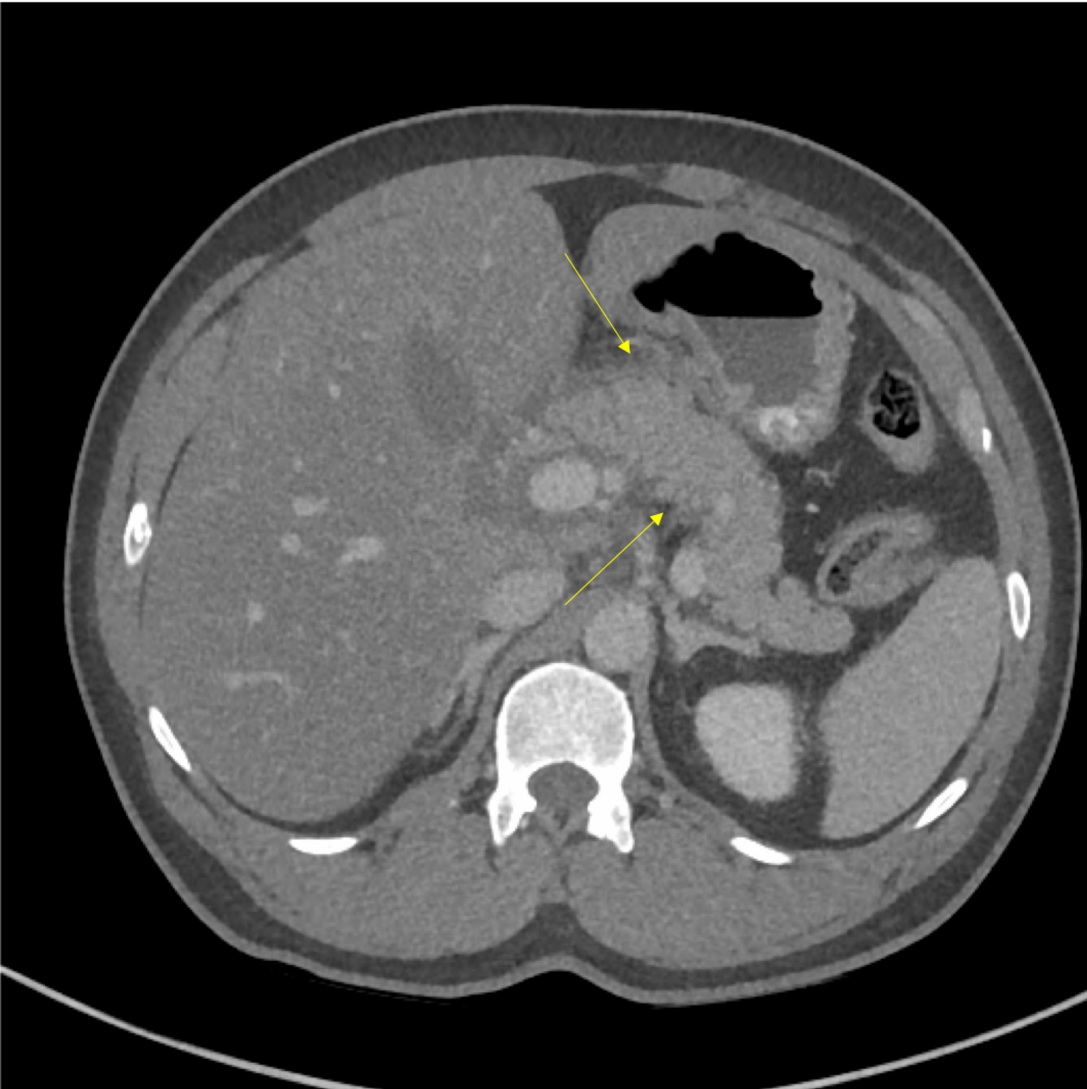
Abdominal CT scan with contrast The image shows non-necrotic pancreatitis (yellow arrows) CT: computed tomography
